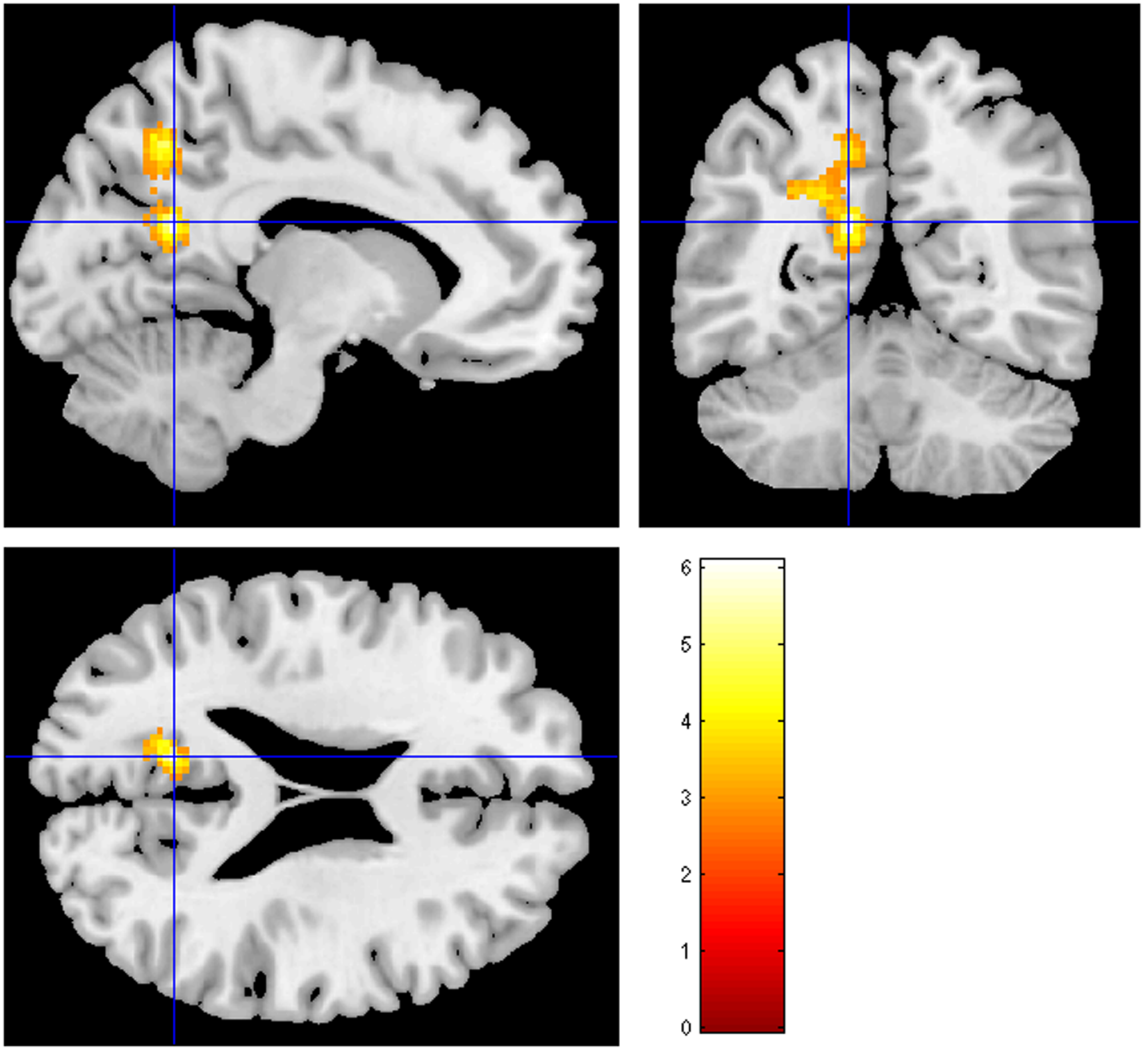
Statistical parametric mapping projections of areas with significantly reduced cerebral perfusion when comparing the follow-up SPECT scans with the initial scans (Uncorrected *p*<0.005). The warm color represents the relative hypoperfusion of the initial scans compared to the follow-up scans.
